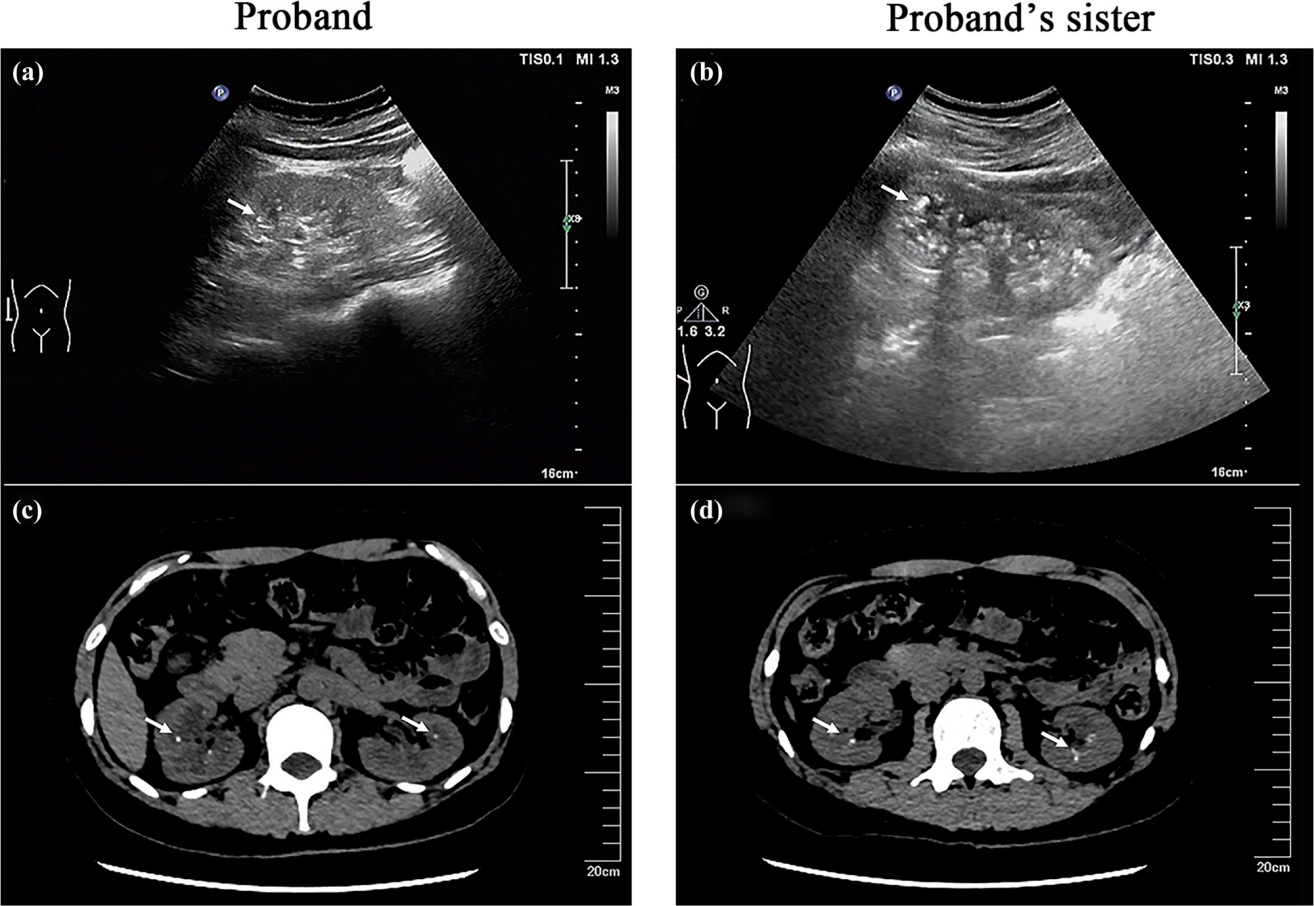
Imaging examinations of the kidneys for the proband and her sister. (a and b) Color Doppler ultrasonography of the kidney of the patient and his sister respectively; (c and d) 16 row spiral Computed Tomography scan images of the patient and her sister respectively. Multiple small stones were found in both kidneys. The arrow designates the stones.
